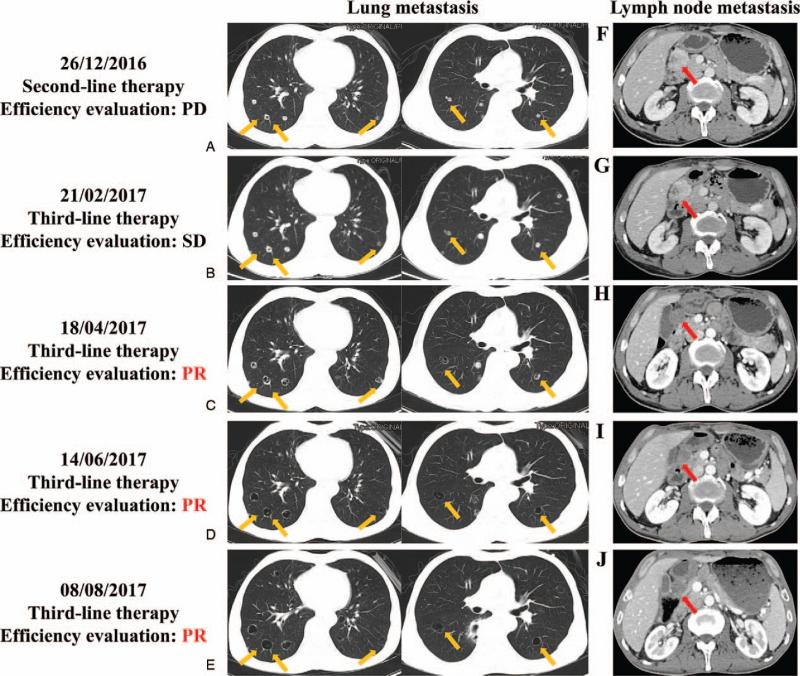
Abdominal and chest enhanced computed tomographic images. (A, B, C, D, E) Lung metastatic nodules (orange arrowhead). (F, G, H, I, J) Lymph nodules in the gastrocolic ligament area (red arrow).
